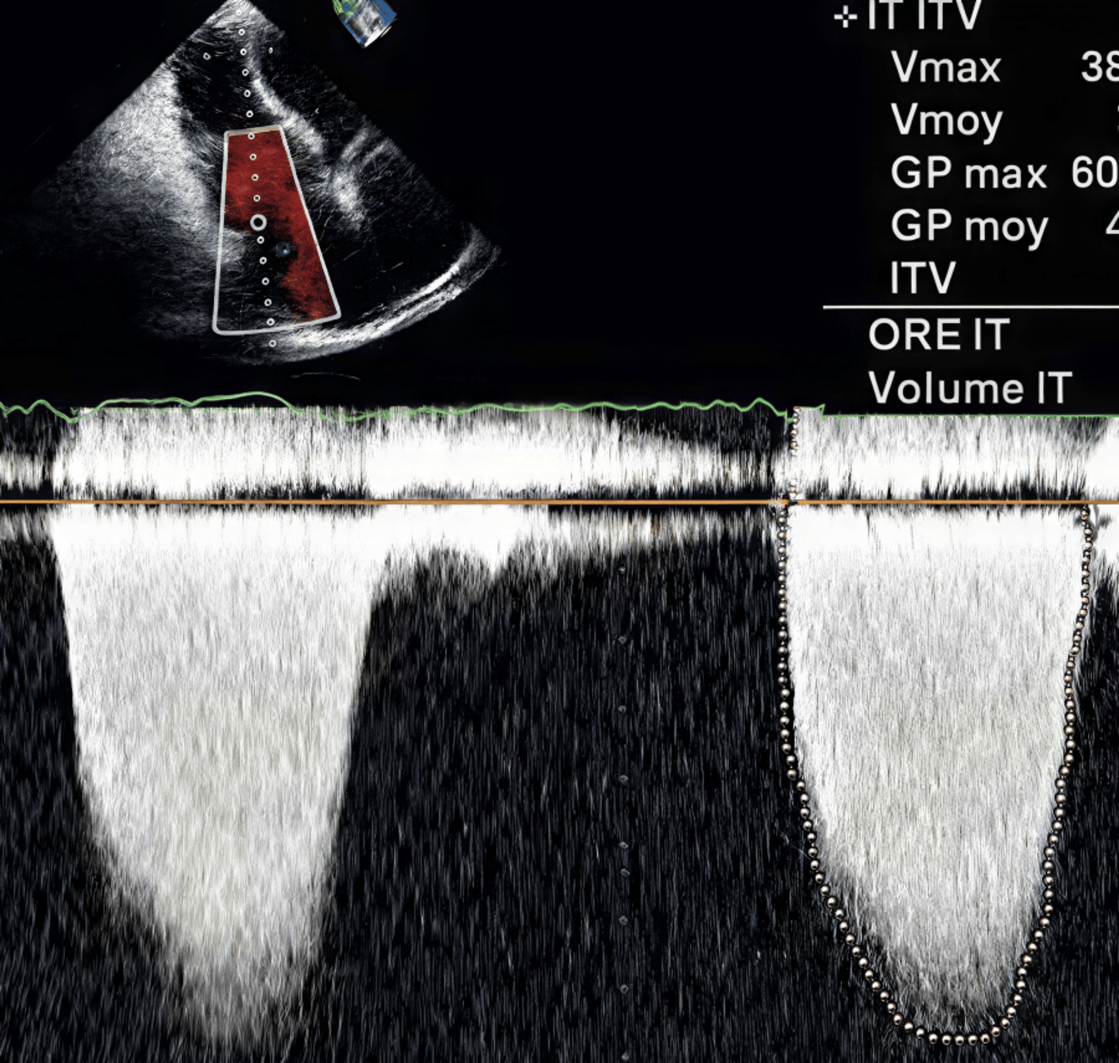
Transthoracic echocardiogram image with pulmonary hypertension This image shows a dilated and hypertrophied right ventricle, moderate mitral regurgitation, and pulmonary hypertension estimated at 70 mmHg.
